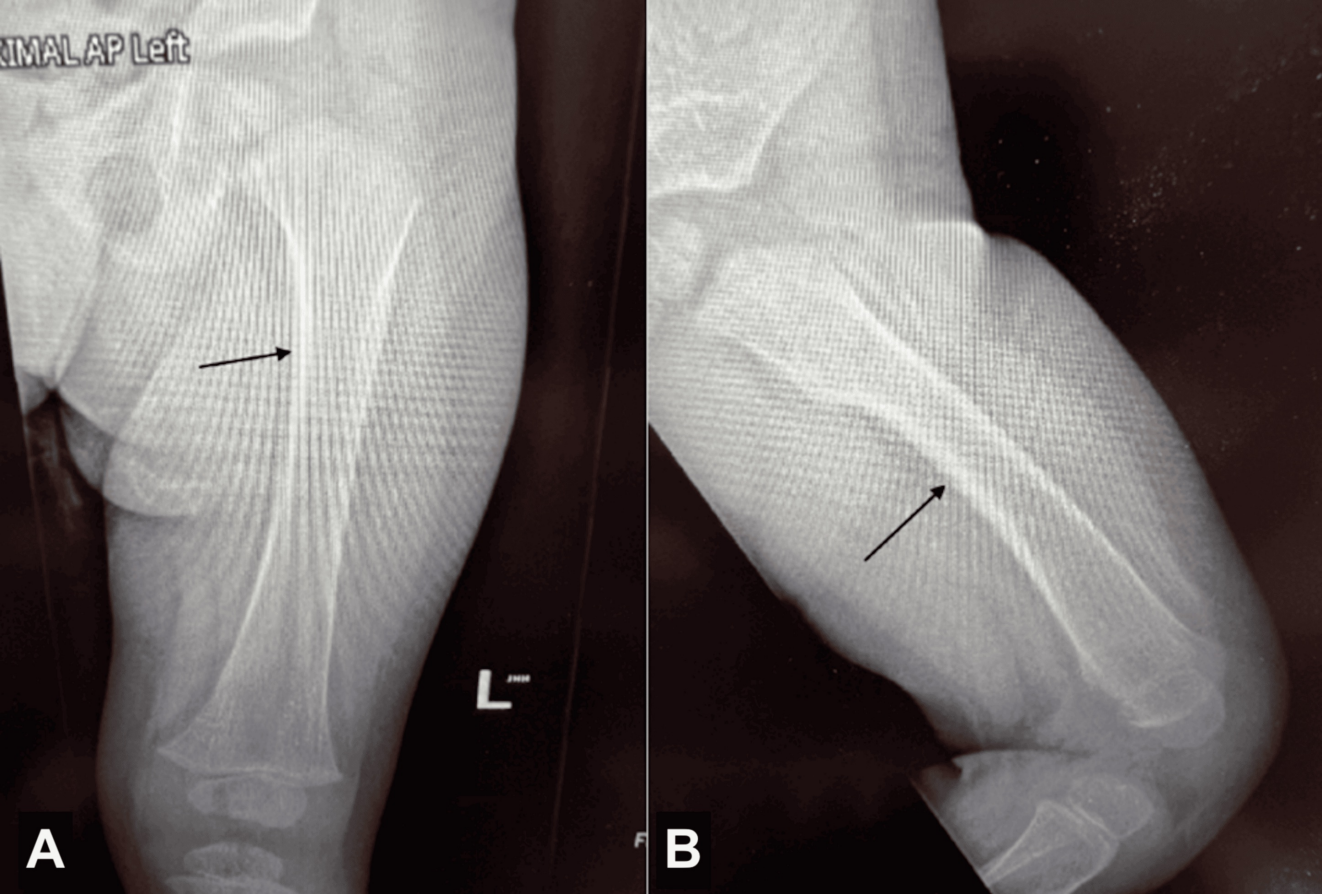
X-rays of the left femur (A) axial and (B) sagittal views. Arrows indicate mild osteopenia, mild bowing, and diaphyseal thickening.
